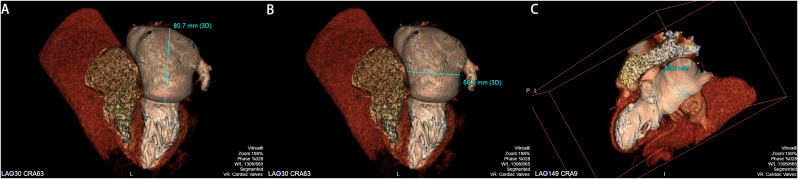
A: Measurement of LA long diameter at the apical four-chamber plane. B: Measurement of LA transverse diameter at the apical four-chamber plane. C: LA anteroposterior diameter measurement along the long axis of the apical two-chamber plane
